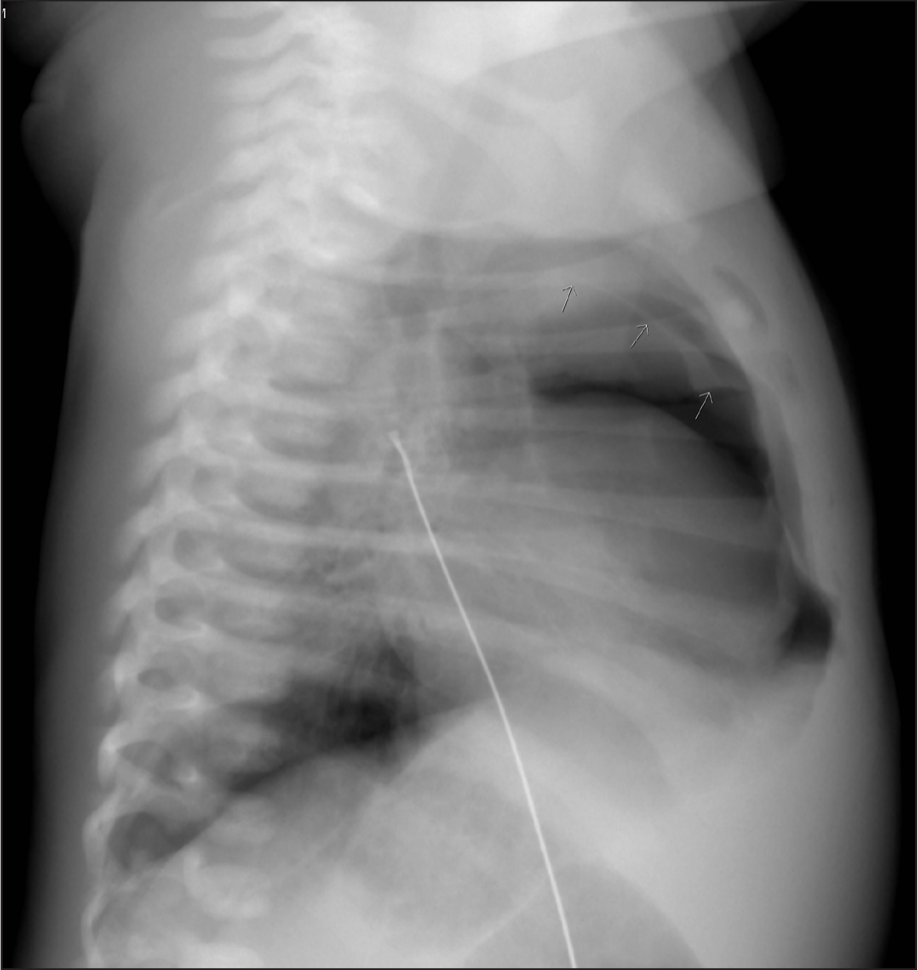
Lateral plain radiograph of the chest. The mediastinal air can be seen as a translucency in the areas surrounding the heart with a sharp delineation.
